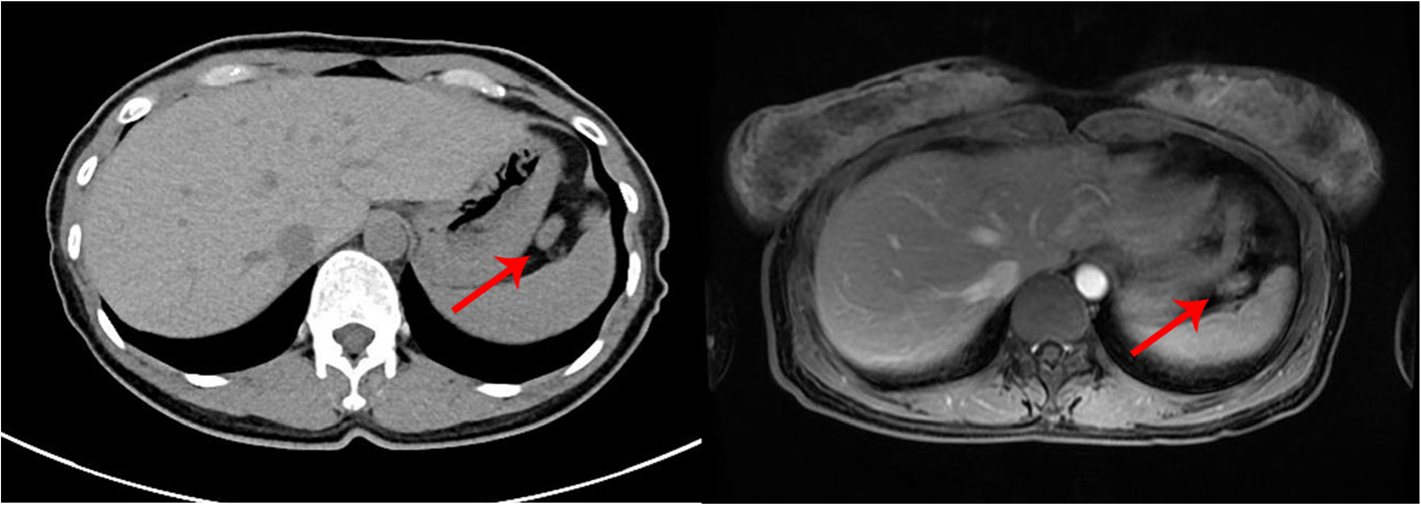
Case 2: enhanced CT (left) and high-resolution MRI (right) of the pancreas showed no obvious mass or high-perfusion nodules within the pancreas, but a nodule was observed above the spleen, which was considered an accessory spleen
